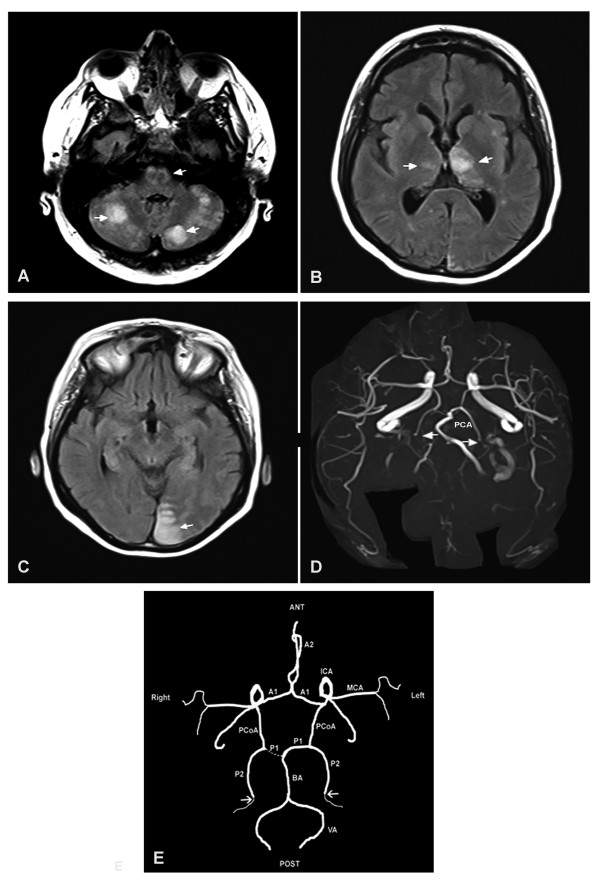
Acute vertebrobasilar territory stroke following percutaneous thromboaspiration of an occluded arteriovenous fistula of the forearm. (A) Magnetic resonance imaging (MRI) of the head. Serial 5 mm axial brain images show the presence of bilateral hypersignal areas (indicated by arrowheads) in the cerebellar hemispheres and bulboprotuberancial junction. (B) Hypersignal in the thalamic areas, predominantly on the left. (C) Hypersignal in the occipital lobe. (D) Polygon of Willis by MRI time of flight show bilateral occlusion of the posterior cerebral arteries. (E) Polygon configuration of Willis in our patient. The arrows show the localization of bilateral occlusion of the Posterior cerebral arteries (PcoA, posterior communicating artery; PCA, posterior cerebral artery (P1 and P2 indicate the PCA segments); ACA, anterior cerebral artery (A1+A2 indicate the ACA segments); MCA, middle cerebral artery; ICA, internal carotid artery; BA, basilar artery; VA, vertebral artery. Note that our patient displays a "fetal type" right PCoA, in that the P1 segment is hypoplastic and the ICA supplies right posterior cerebral territory via PCoA.
